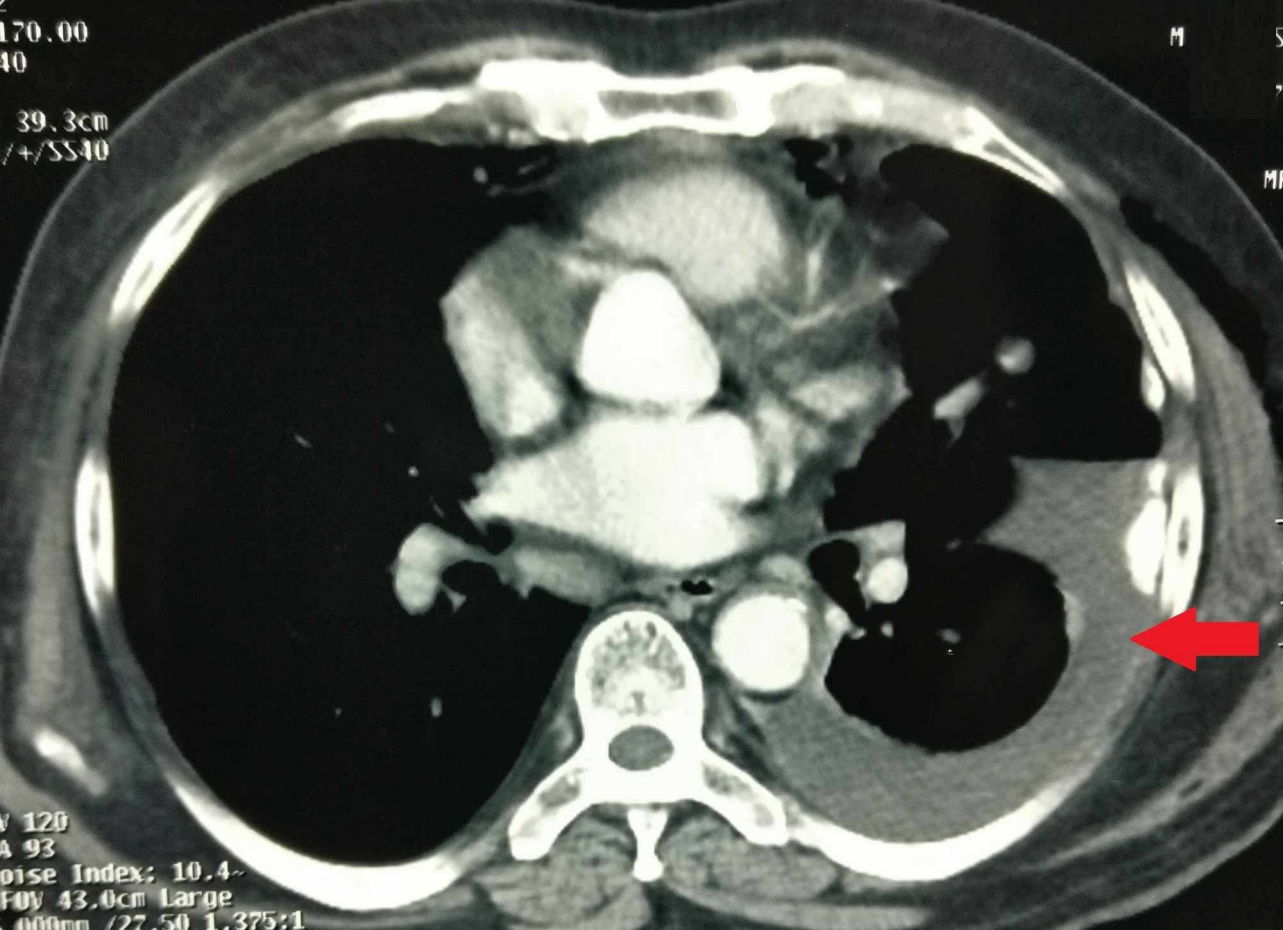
Contrast enhanced CT scan of chest showing left side pleural effusion, hydropneumothorax with resultant partial collapse of left lung, left lung pleural thickening with calcification and a pleural-based nodule measuring 17 x 13 mm in left upper lung.
